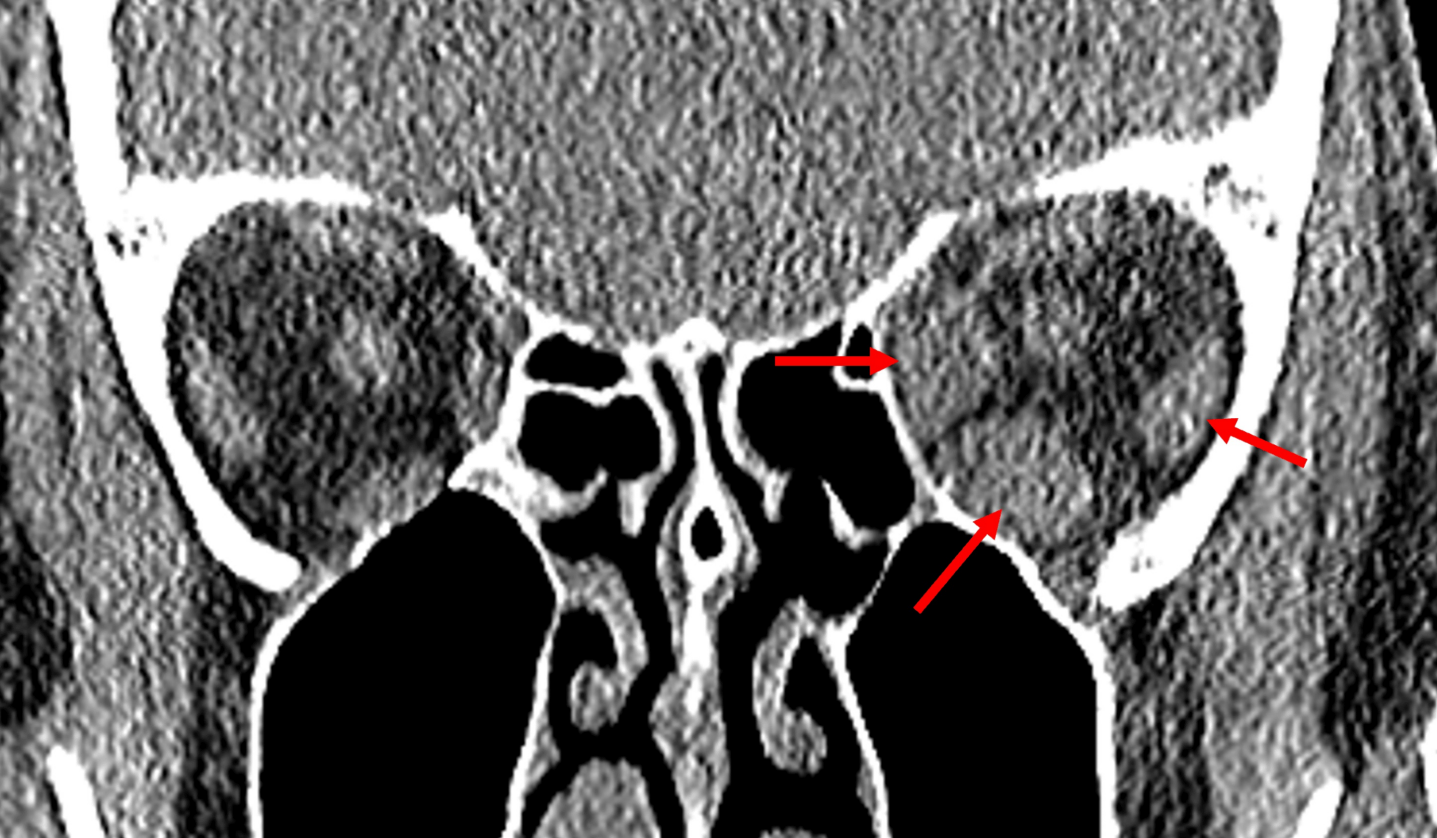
CT sinuses without contrast showing enlarged left medial, inferior, and to a lesser extent lateral rectus extraocular muscles (arrows) consistent with TED CT: computed tomography, TED: thyroid eye disease
